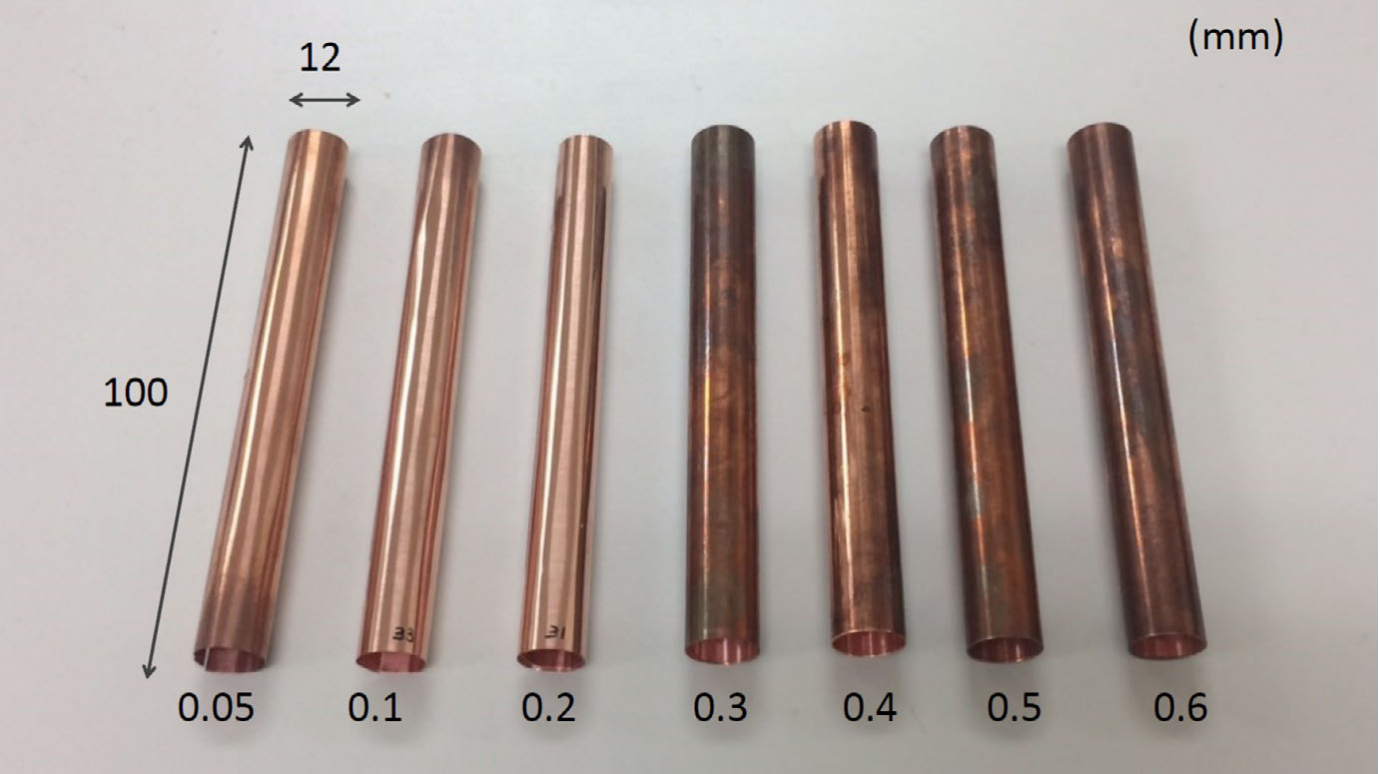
Fabricated copper pipes for measuring the half‐value layer in a CT scanner.
